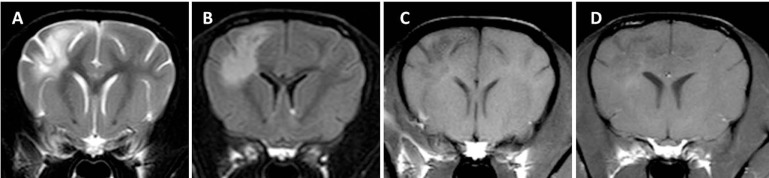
Transverse magnetic resonance images (3 T) of a Chihuahua (11 months, female) with necrotizing meningoencephalitis. T2 (A) and fluid-attenuated inversion recovery (B) hyperintense finger-like lesions affecting the subcortical white and gray matter in the right prosencephalon. These characteristics may resemble those of Yorkshire Terriers with necrotizing leucoencephalitis since there might be a thin rim of intact cortical gray matter. However, involvement of gray matter can be visualized in most cases. The lesion is hypointense on T1-weighted images (C) and is only mildly enhanced in the periphery of the lesion (D).
